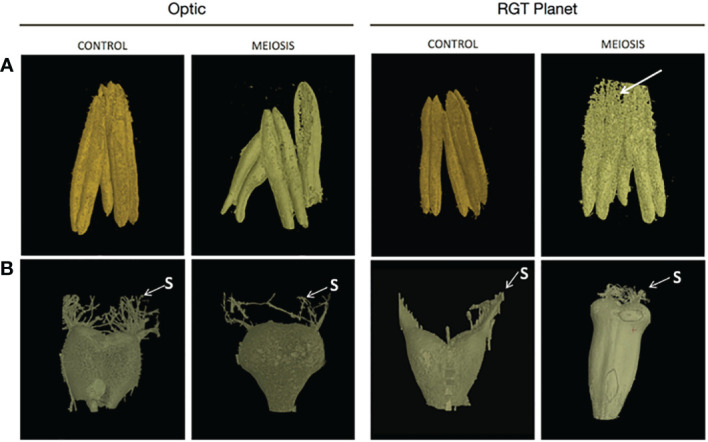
X-ray CT images of (A) anther and (B) carpel development from a representative floret from control and heat stress conditions for barley cultivars Optic and Planet. X-ray images of the anthers and carpels obtained by X-ray CT were compared for control environment and heat stress environment (heat stress during meiosis of pollen development) immediately before anthesis. Arrow indicates the broken septum in RGT Planet heat stressed anther.
